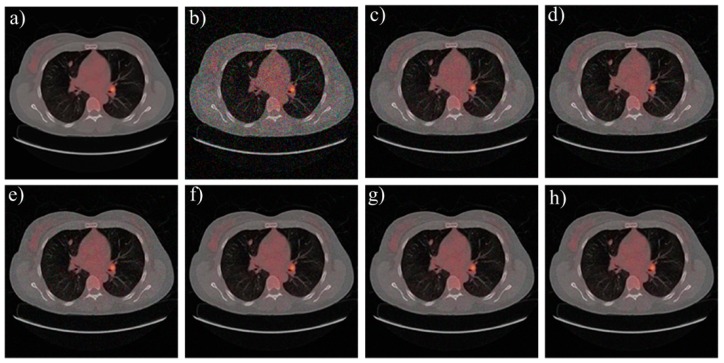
Denoising performance of the six algorithms to the CT image. (a) Original image; (b) noisy image; (c) MP; (d) OMP; (e) BP; (f) FAL0; (g) AL0; (h) AL0-L.
